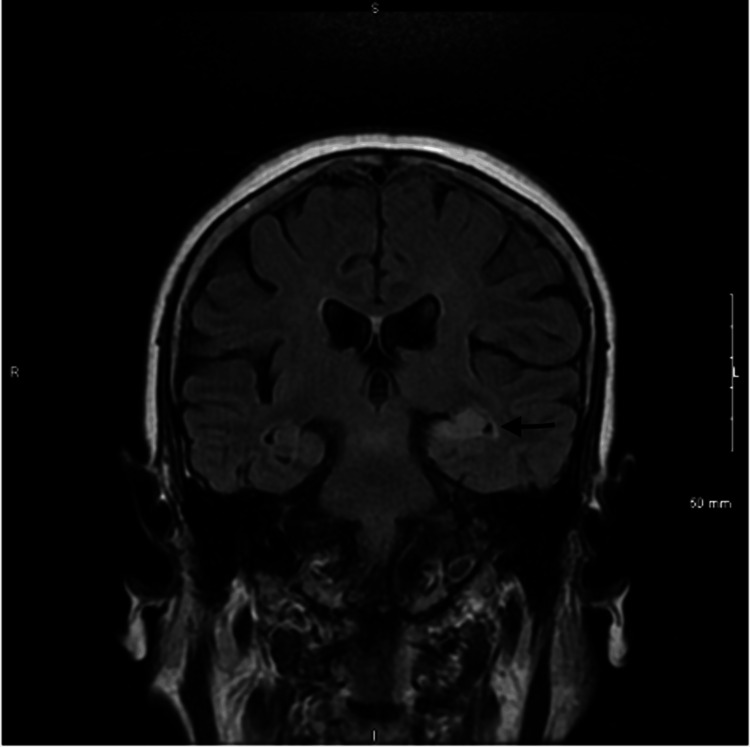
This coronal view of brain MRI FLAIR with no contrast shows an increased T2 signal that involves the left hippocampus and extends posteriorly. MRI: magnetic resonance imaging; FLAIR: fluid-attenuated inversion recovery
